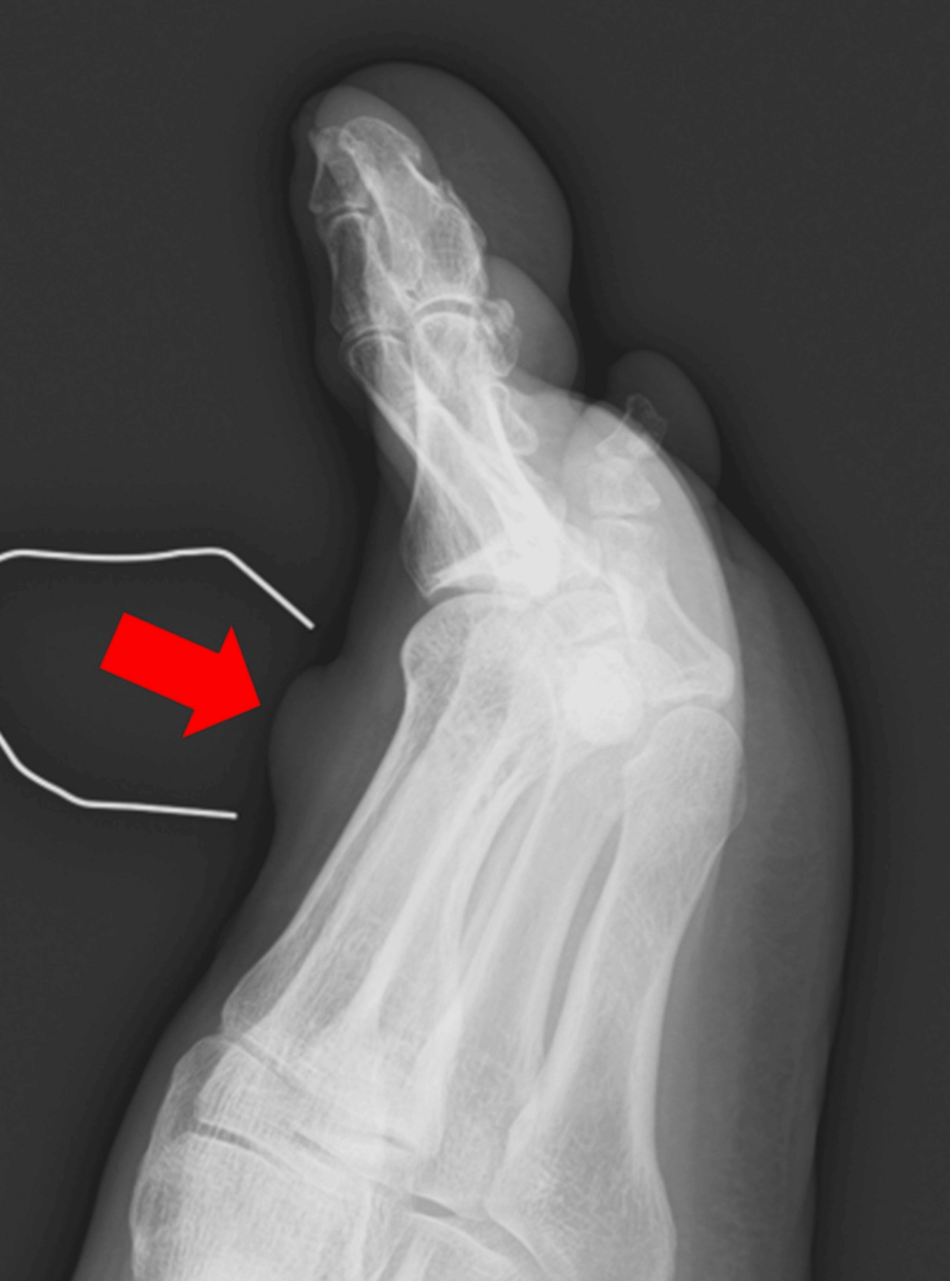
Plain radiography No soft tissue calcifications or abnormalities in the surrounding bone were observed. The white lines represent the metal marker used during the X-ray examination
